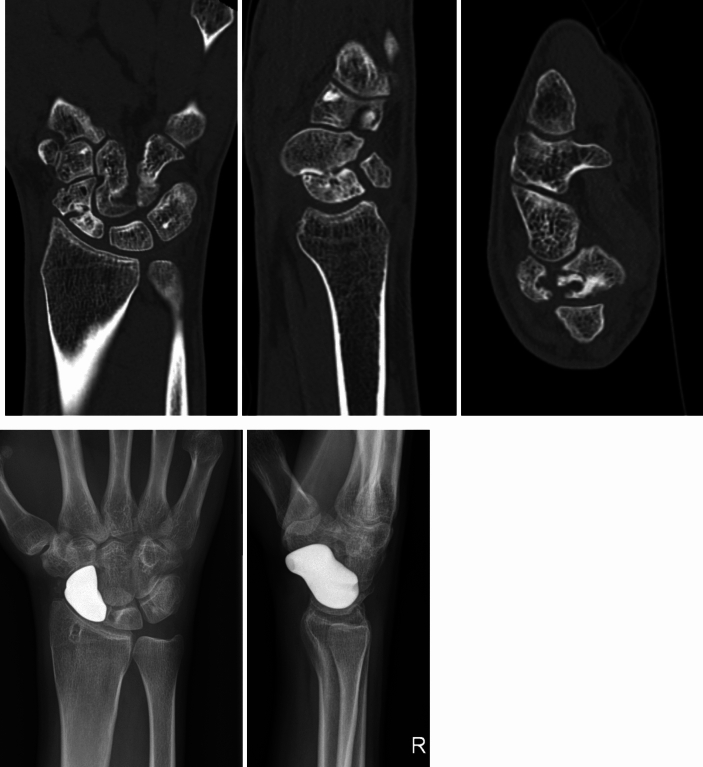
CT-scans of a complex scaphoid non-union 6 years after initial trauma and implanted scaphoid prosthesis
